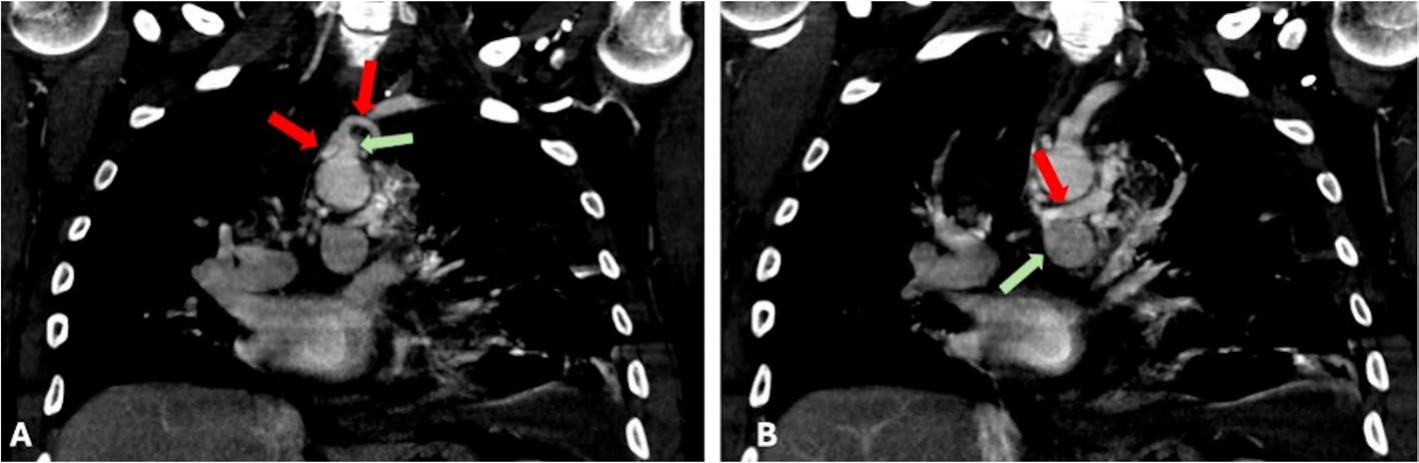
A Coronal image of CT chest with contrast demonstrating the two feeding arteries (red arrows) arising from the proximal left subclavian artery. Their common trunk is noted by the green arrow. B Coronal image of the CT chest with contrast demonstrating the draining vein (red arrow) connecting to the proximal left main pulmonary artery (green arrow)
